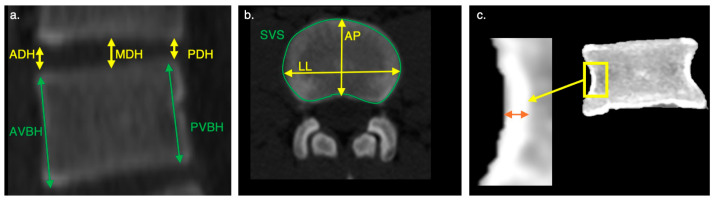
Measurements taken on the vertebrae CT images. ADH = anterior disc height, MDH = middle disc height, PDH = posterior disc height, AVBH = anterior vertebral body height, PVBH = posterior vertebral body height (a); AP = anterior–posterior (dorsoventral) discal surface length (body length), LL = lateral–lateral (right–left) discal surface breadth (body breadth), SVS = superior vertebral body surface area (encircled by green line) (b); cortical shell thickness (orange arrow), measured on mid-coronal section of the vertebral body at mid-height (yellow box) (c).
